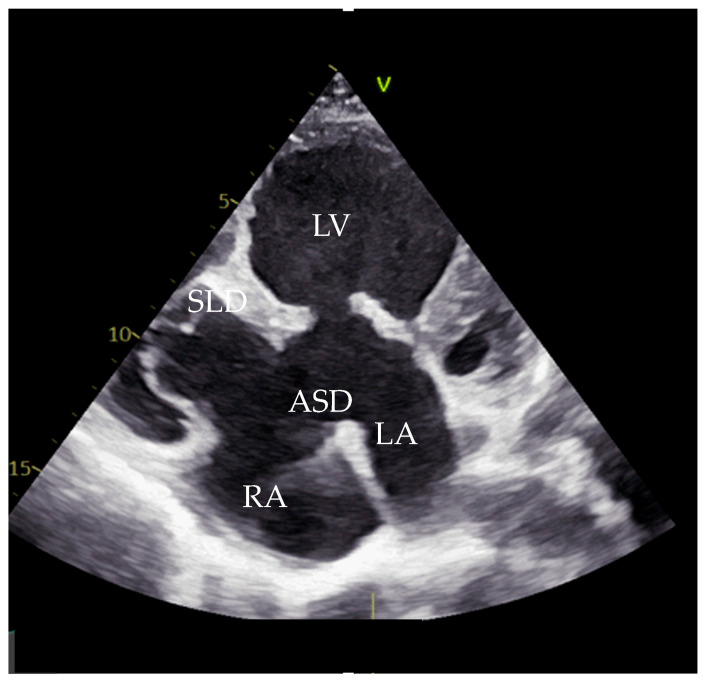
Echocardiography with apical four-chamber view showing apical displacement of tricuspid septal leaflet. LV—left ventricle, LA—left atrium, RA—right atrium, ASD—atrial septal defect, SLD—septal leaflet displacement.
